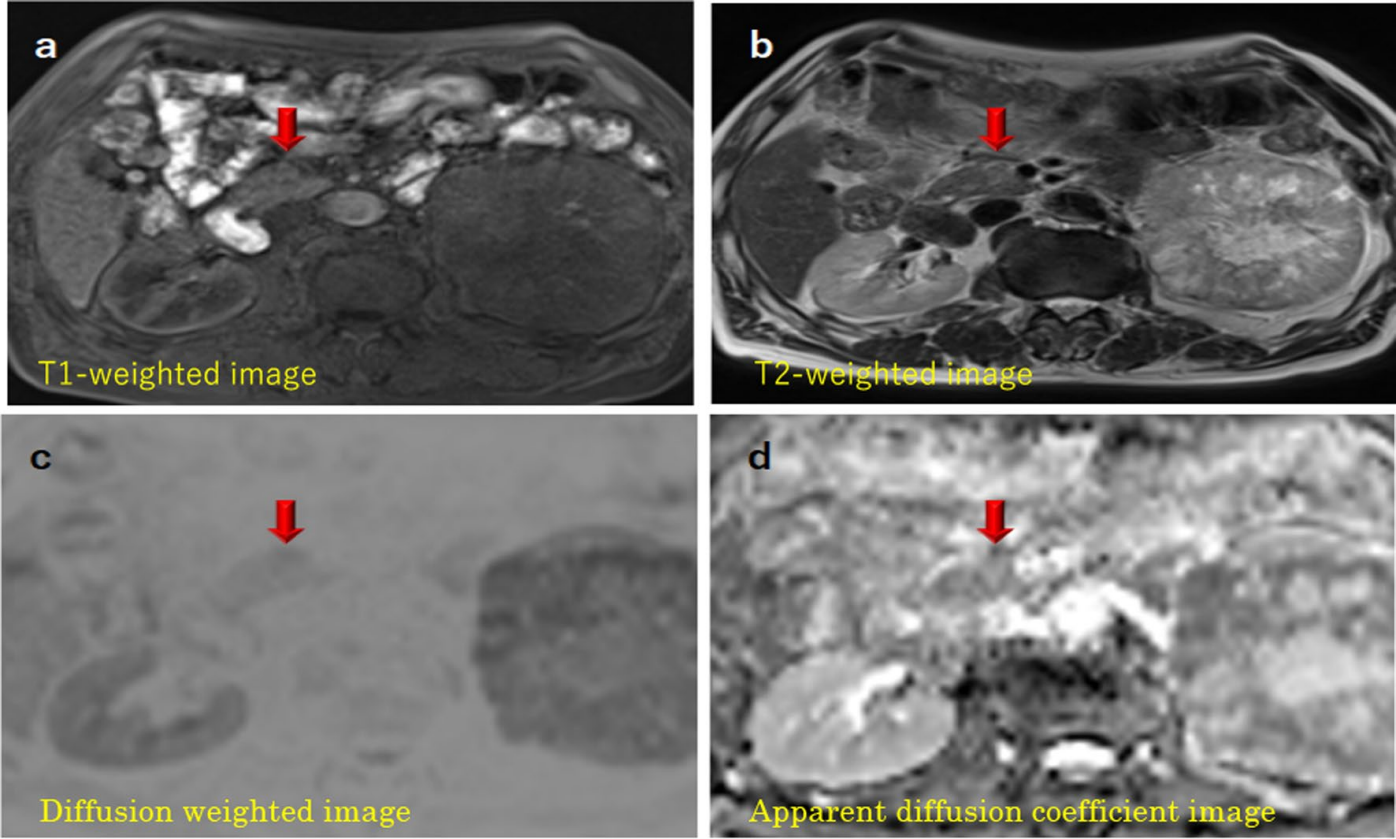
Gd-DTPA-enhanced MRI findings of the pancreatic head tumor. The pancreatic tumor presented with homogenous hypointensity on a T1-weighted imaging and b T2-weighted imaging. c The pancreatic tumor presented with mild hyperintensity on diffusion-weighted imaging. d The aforementioned hypointensity was unclear on apparent diffusion coefficient mapping
